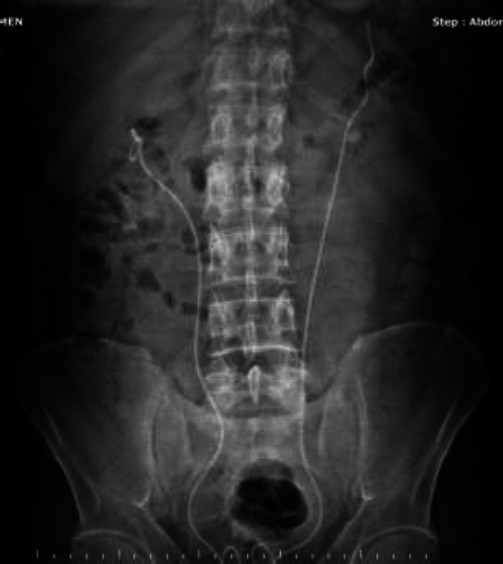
KUB radiography of Patient 1 taken on the 1st postoperative day.
